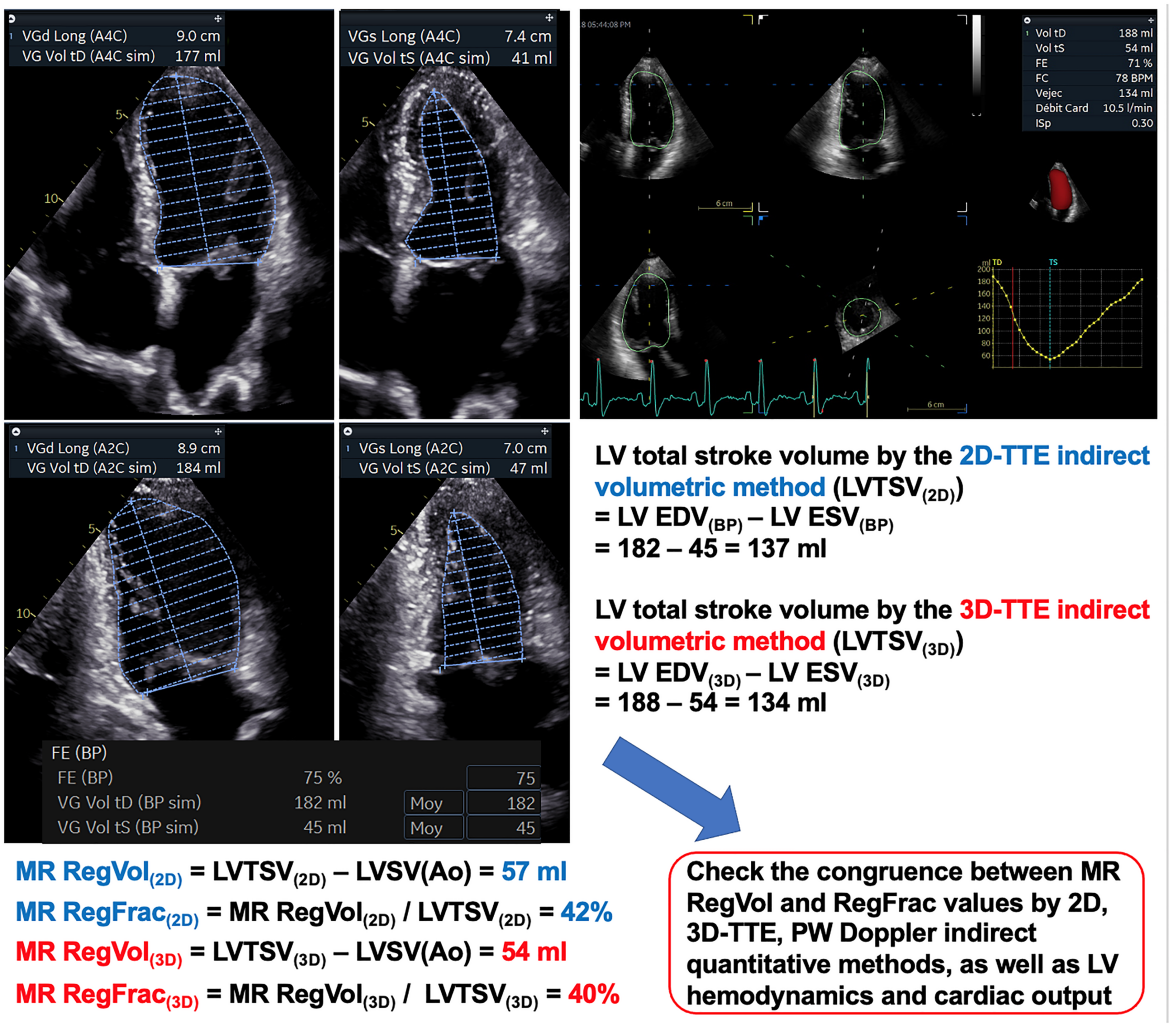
Indirect volumetric method. Same patient as Figures 5, 7. The LV total stroke volume is computed as the difference between LV end-diastolic and end-systolic volumes according to the 2D-biplane Simpson's method [LVTSV_(2D)_] or 3D-volumes [LVTSV_(3D)_]. The LV borders should be traced at the interface between the LV cavity and the compacted myocardium, and not at the blood-tissue interface (that is at the tip of the trabeculation). The aortic systolic forward stroke volume is computed by the Doppler method (see Figure 7). The MR-RegVol_(2D, 3D)_ is computed as the difference between the LVTSV_(2D, 3D)_ and the LVSV(Ao). The MR-RegFrac_(2D, 3D)_ is obtained by dividing the MR RegVol_(2D, 3D)_ by the LVTSV_(2D, 3D)._ The congruence between MR RegVol and RegFrac values obtained by the different indirect quantitative methods as well as LV hemodynamic and cardiac output should be carefully checked. Δ, diameter; BP, biplane; EDV, end-diastolic volume; ESV, end-systolic volume; LV, left ventricular/ventricle; LVSV(Ao), LV systolic aortic forward stroke volume by Doppler method; LVTSV_(2D)_, LV total stroke volume by 2D-indirect volumetric method; LVTSV_(3D)_, LV total stroke volume by 3D-indirect volumetric method; MR, mitral regurgitation; RegVol, regurgitant volume; RegFrac, regurgitant fraction.
